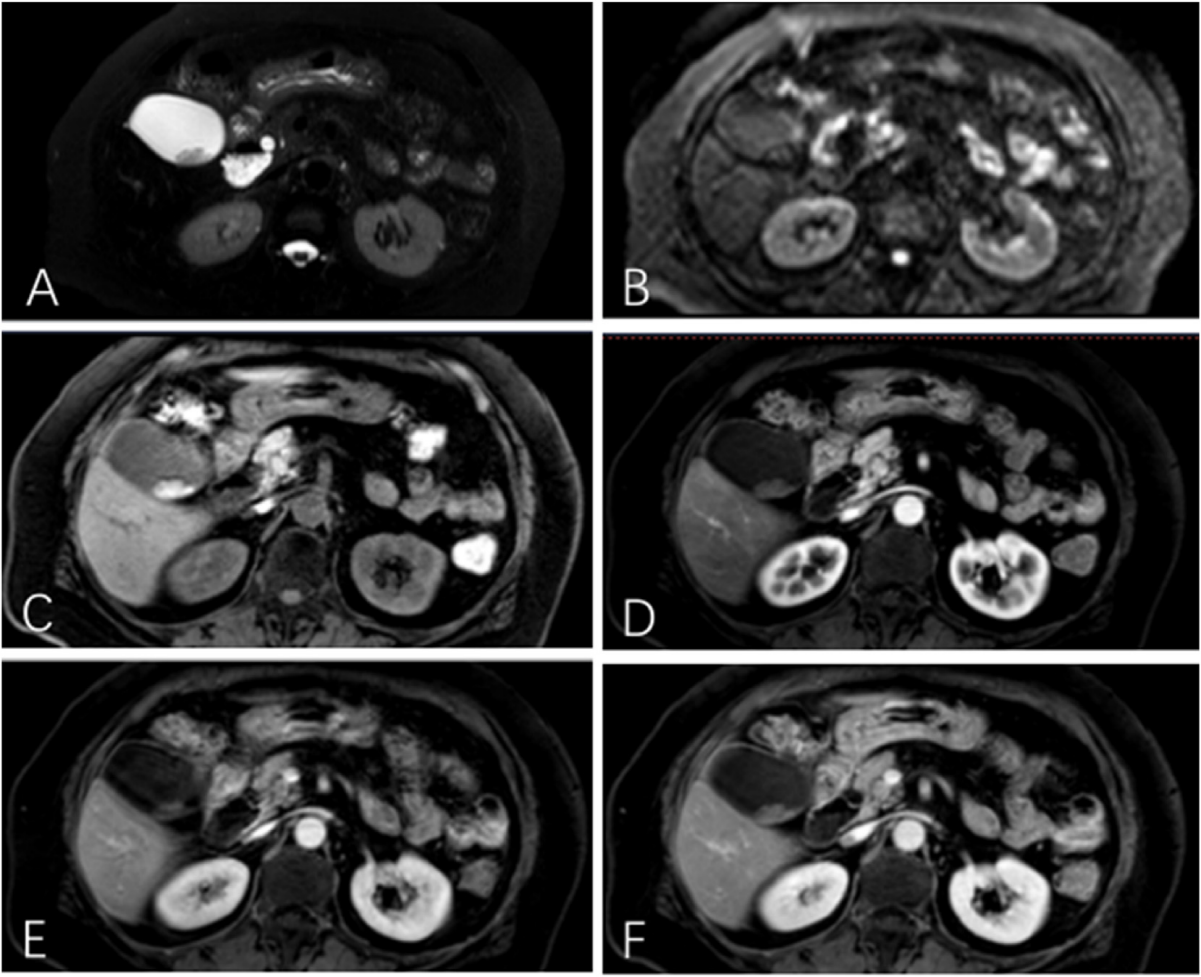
MRI features of gallbladder adenoma from Patient No 3. Axial T2-weighted (A) and T1-weighted (C) images show marked asymmetric mural thickening that is moderately hyperintense without diffusion restriction (B), and shows poor contrast enhancement in artery, portal, and vein phase (D–F). The tumor size was 2.4 cm times 1.1 cm.
